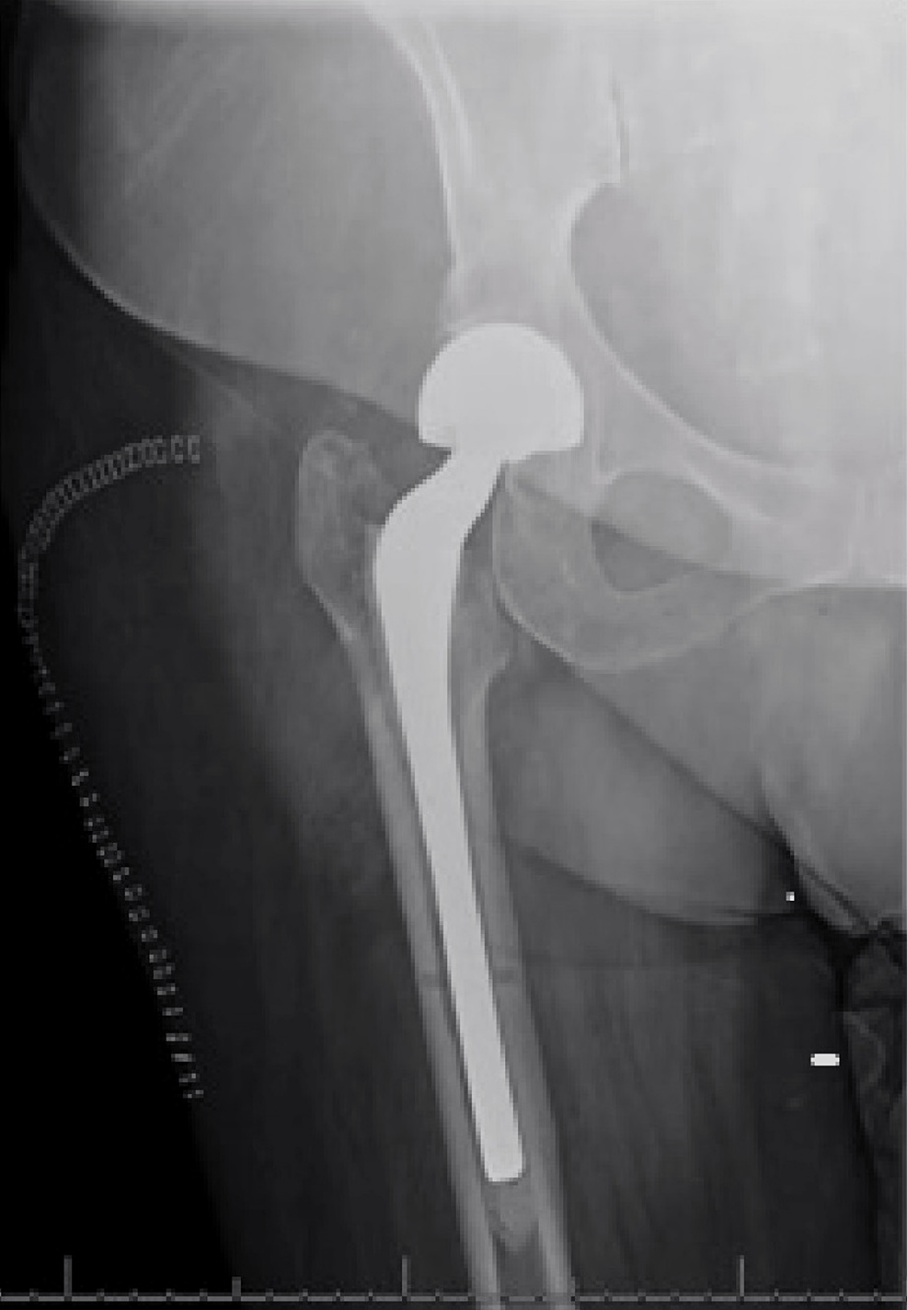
AP radiograph of the right hip one day prior to having increased hip pain shows the PROSTLALAC spacer in place without any evidence of loosening or acute fracture. AP - anterior-to-posterior
